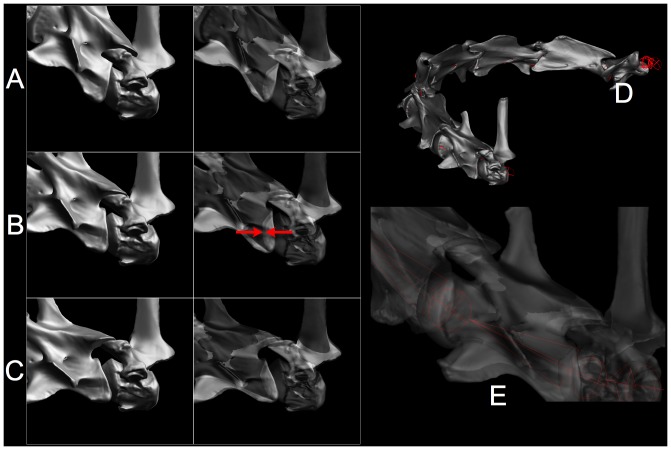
Digital articulation. CT data of individual vertebrae of a recent giraffe Giraffa Camelopardalis are articulated in Autodesk Maya [108]. Cervical vertebra C7 pivots about a center of rotation that closely corresponds to the center of curvature of the roughly hemispherical condyle of T1, confirmed by exploratory manipulation and adjustment, resulting in close intervertebral separations as reported in [15] (see red arrows). In A–C, by alternating between opaque and transparent one can observe osteological bracing dorsiflexion (A) and the ZSF at the limit of ventriflexion. With all intervertebral joints adjusted (D–E), the articulated neck approximates the range of motion observed in life (see also Figures 11, 12). This method applies equally to the similarly opisthocoelous vertebrae [30]–[32], see Figure 25. CT data provided courtesy American Museum of Natural History.
